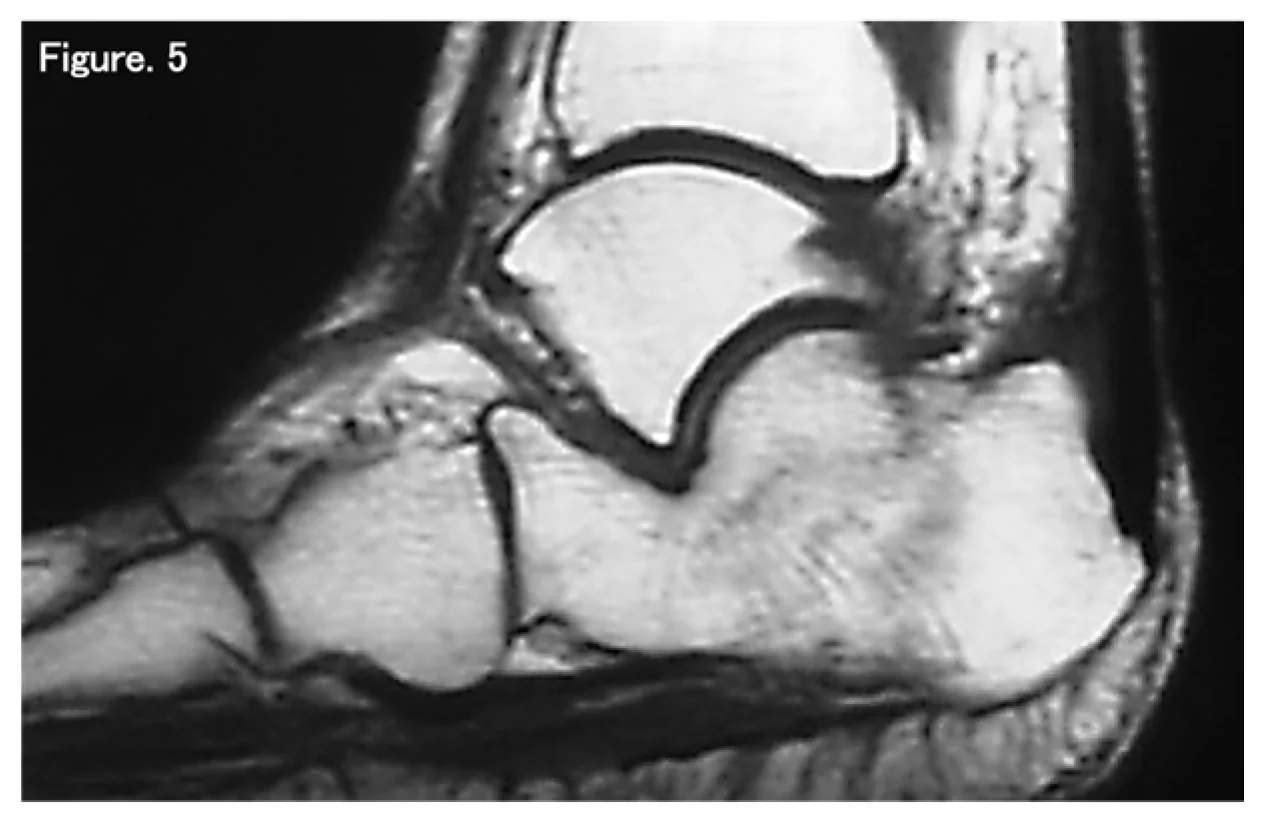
Sagittal T1-weighted MRI of an 86-year-old woman showing a linear low-signal lesion in the calcaneus.
